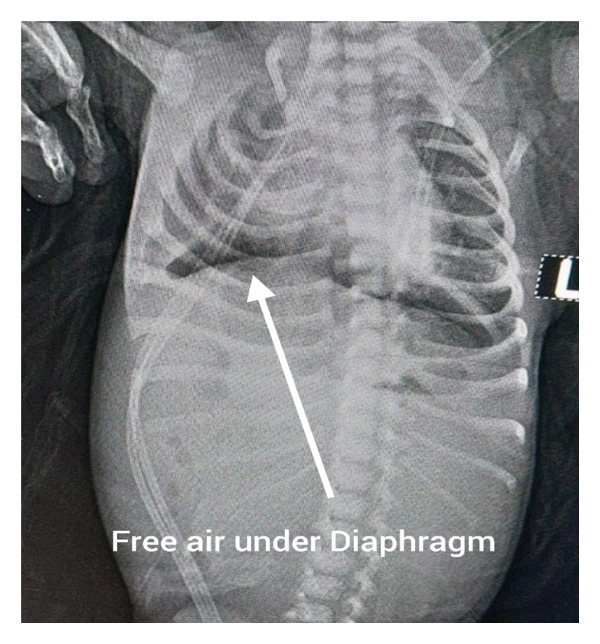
Erect abdominal radiograph demonstrating pneumoperitoneum. The arrows highlight free subdiaphragmatic air under both hemidiaphragms, consistent with intestinal perforation.
